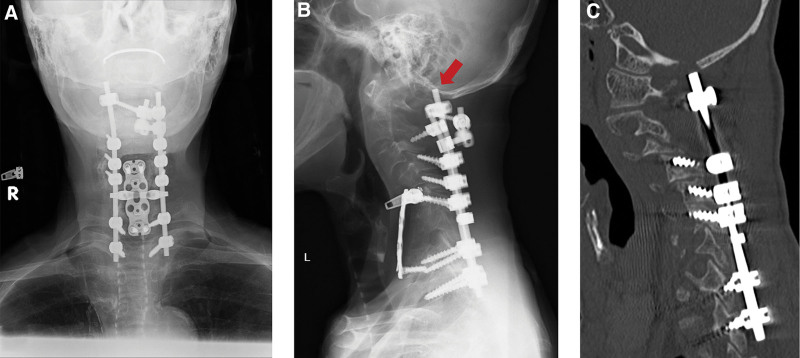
Cervical spine X-ray and computed tomography (CT) after 6 months of posterior fusion. (A) The anteroposterior view. The arrow shows the occipital bone erosion; (B, C) the lateral view.
